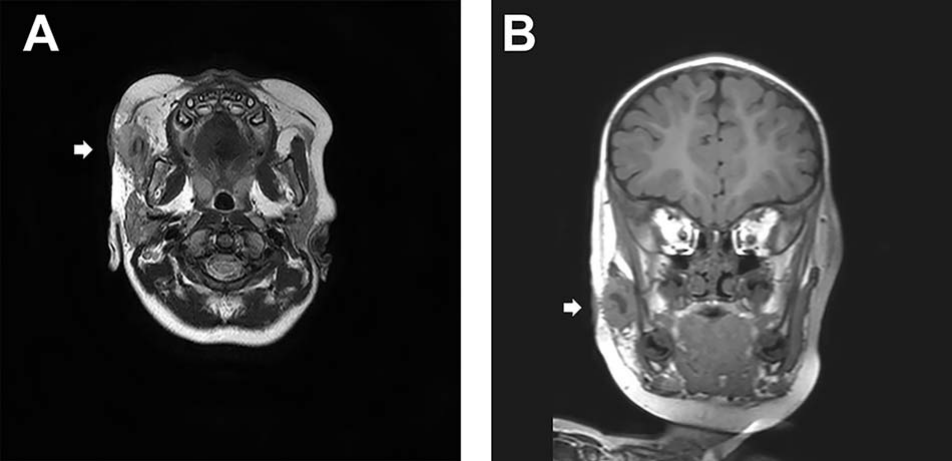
(A) A transverse sT2W mDixon MRI image. A cystic lesion beneath the superficial musculo-aponeurotic system is identified. (B) A coronal section of MRI.
